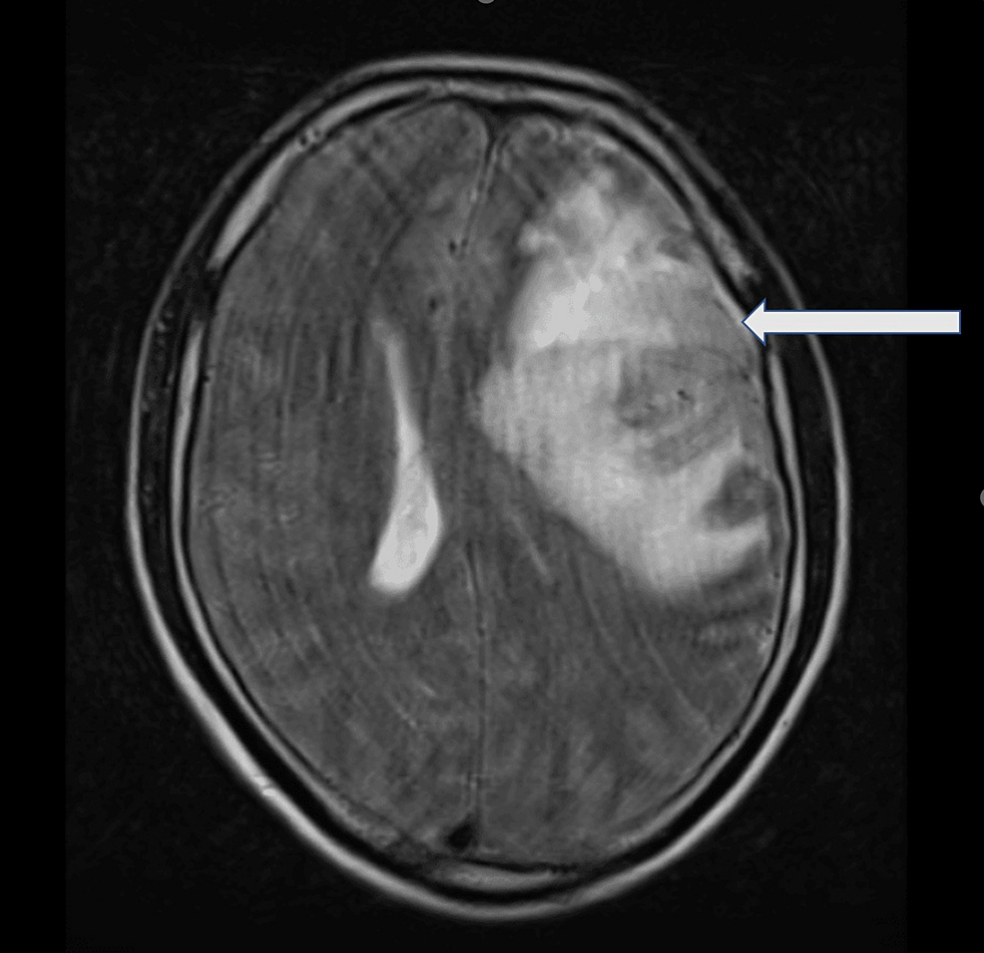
T2-weighted magnetic resonance imaging of the brain. The magnetic resonance imaging shows hyperintensity in the left middle cerebral artery territory suggestive of an acute infarct.
